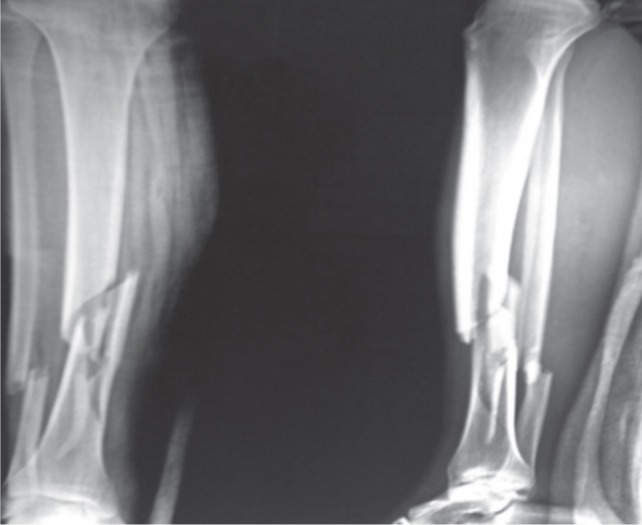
Radiograph showing the fracture of tibia and fibula at junction of proximal two-third and distal one-third.
